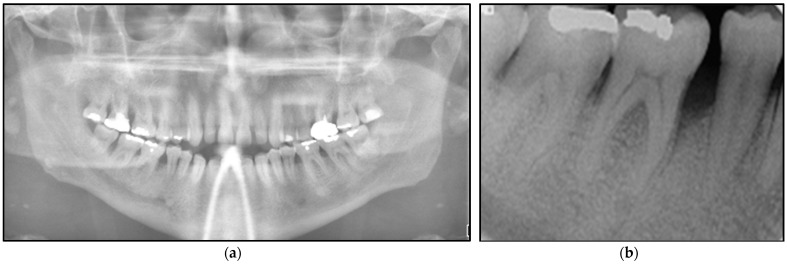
Shows the radiographic investigations. (a) represents the orthopantomogram imaging (extraoral) was taken in order to determine and evaluate the level of bone loss in the area of lower right 2nd premolar (LR5) and 1st molar (LR6) where the intraoral lesions presented compared with the contralateral side. It shows generalised bone loss without involving the bifurcation among the lower molars. (b) represents along-cone intraoral periapical view showing vertical bone loss distal to the LR5 and mesial to the LR6, whereby the intraoral lesion was presented intraorally. The evidence of bone loss is an indicative of the extension of the lesion beyond the soft tissue reaching the periosteum and alveolar bone. Otherwise, both images show no pathology.
